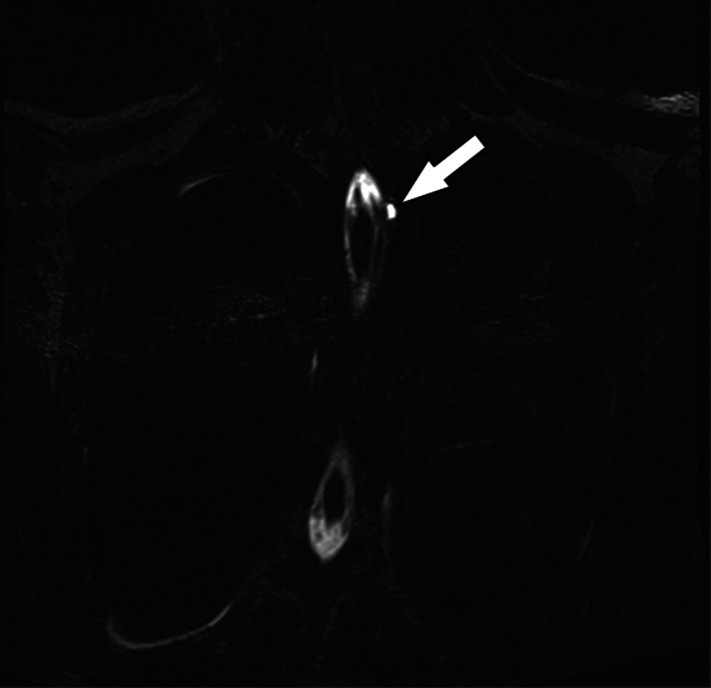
Magnetic resonance imaging (coronal view) showing a meningeal diverticulum at the first and second thoracic level (arrow).
